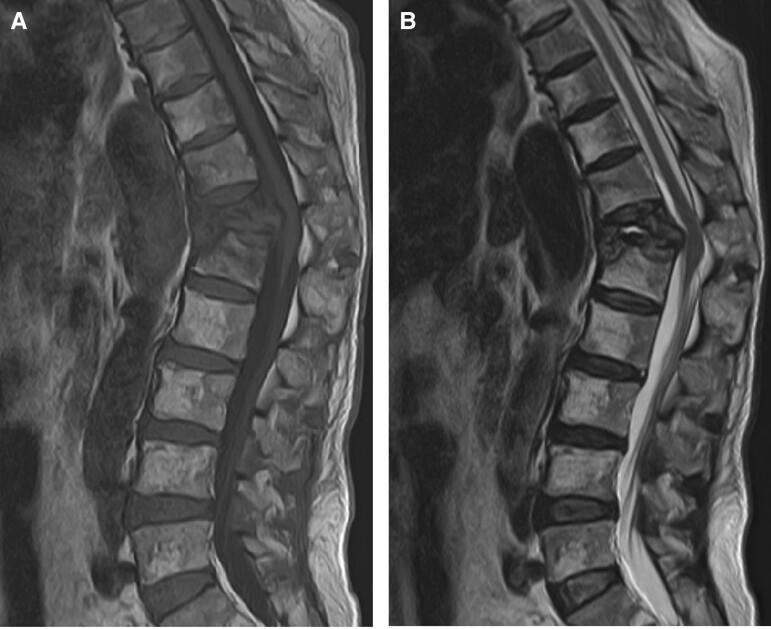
Magnetic resonance imaging showed the T12–L1 vertebral fractures and their advance into the spinal canal. *A*, Sagittal, T1-weighted. *B*, Sagittal, T2-weighted.
